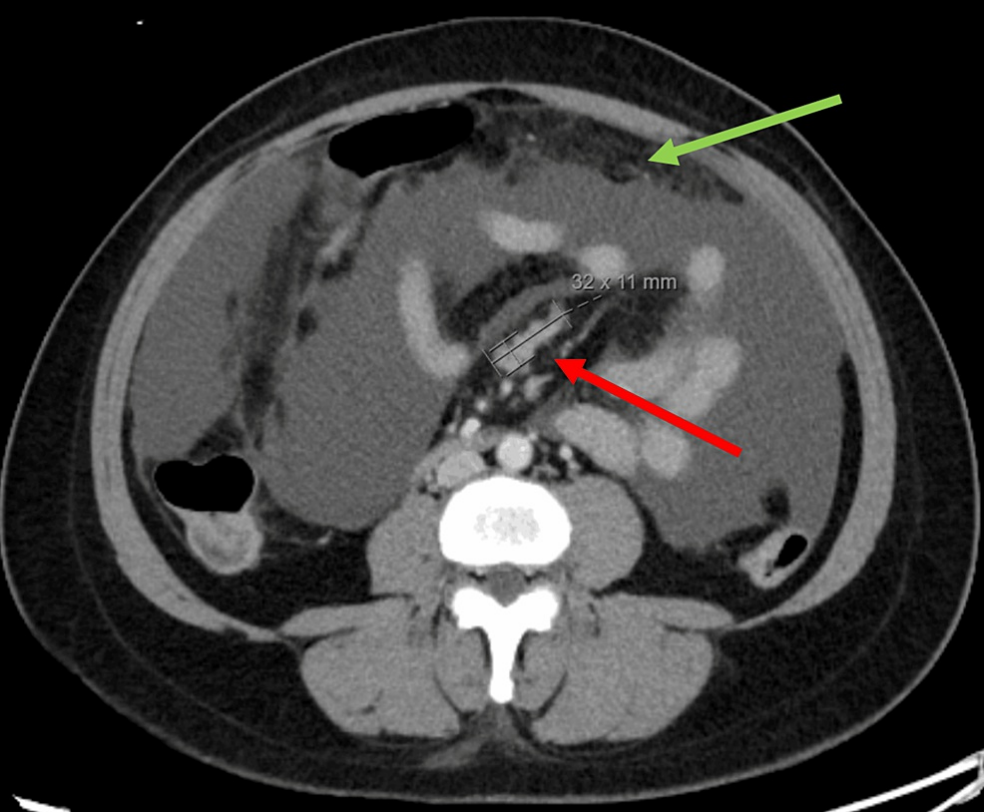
CT of the abdomen/pelvis demonstrating ascites, omental stranding (green arrow), and lymphadenopathy (red arrow). CT: computed tomography
